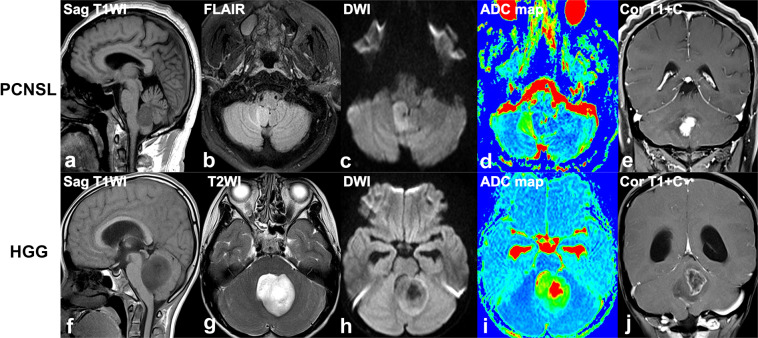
(a–e). A 52-year-old man with a diffuse large B cell lymphoma in right cerebellum, presenting with intermittent dizziness. (f–j) A 11-year-old girl with a anaplastic astrocytoma in vermis region of cerebellum, presenting with headache, dizziness, nausea and vomiting for two weeks. Sagittal T1W (a,f), axial fat-saturated FLAIR (b) and T2W (g), diffusion-weighted (b = 1000 sec/mm^2^) (c,h), apparent diffusion coefficient (ADC) map (d,i), coronal contrast-enhanced T1W (e,j) images reveal a homogeneously enhancing tumor in the right cerebellar vermis region (a–e), with mild streak-like edema (b), diffusion restriction with low ADC value (c,d), and branch-like enhancement (e), and a heterogeneously enhancing tumor in cerebellar vermis region (e-j), with mild edema (f), hypo- in centre and iso-intensity diffusion signal in periphery of tumor with relatively high ADC value (h,i), eccentric cystic change and peripheral ring-like enhancement (h).
